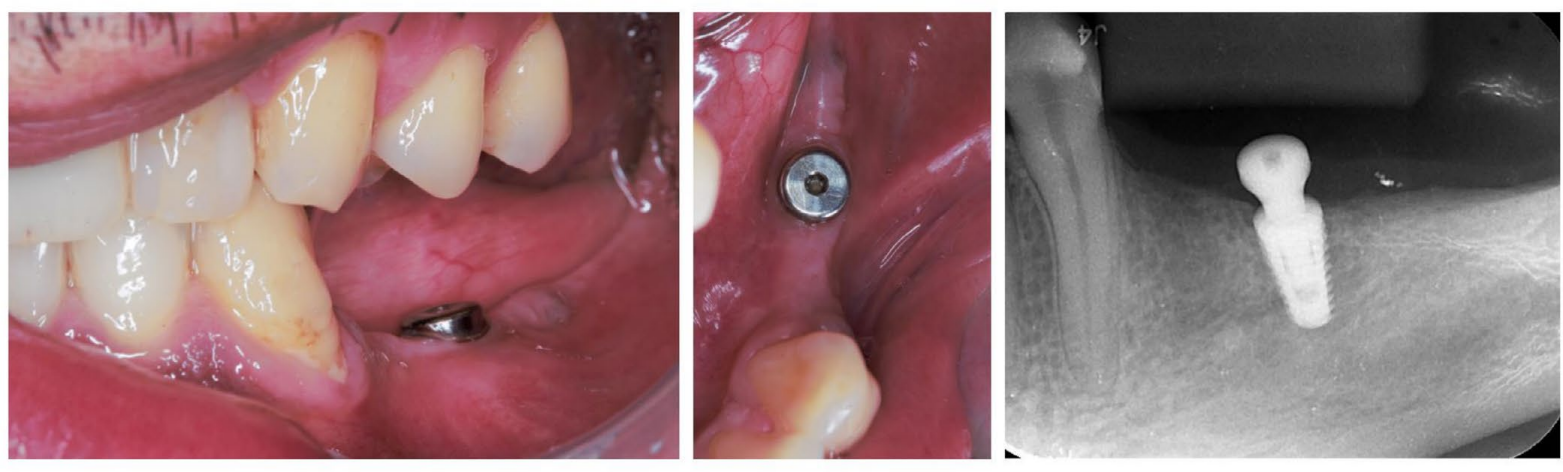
Clinical and radiographic aspects in the 2‐week recall visit.
